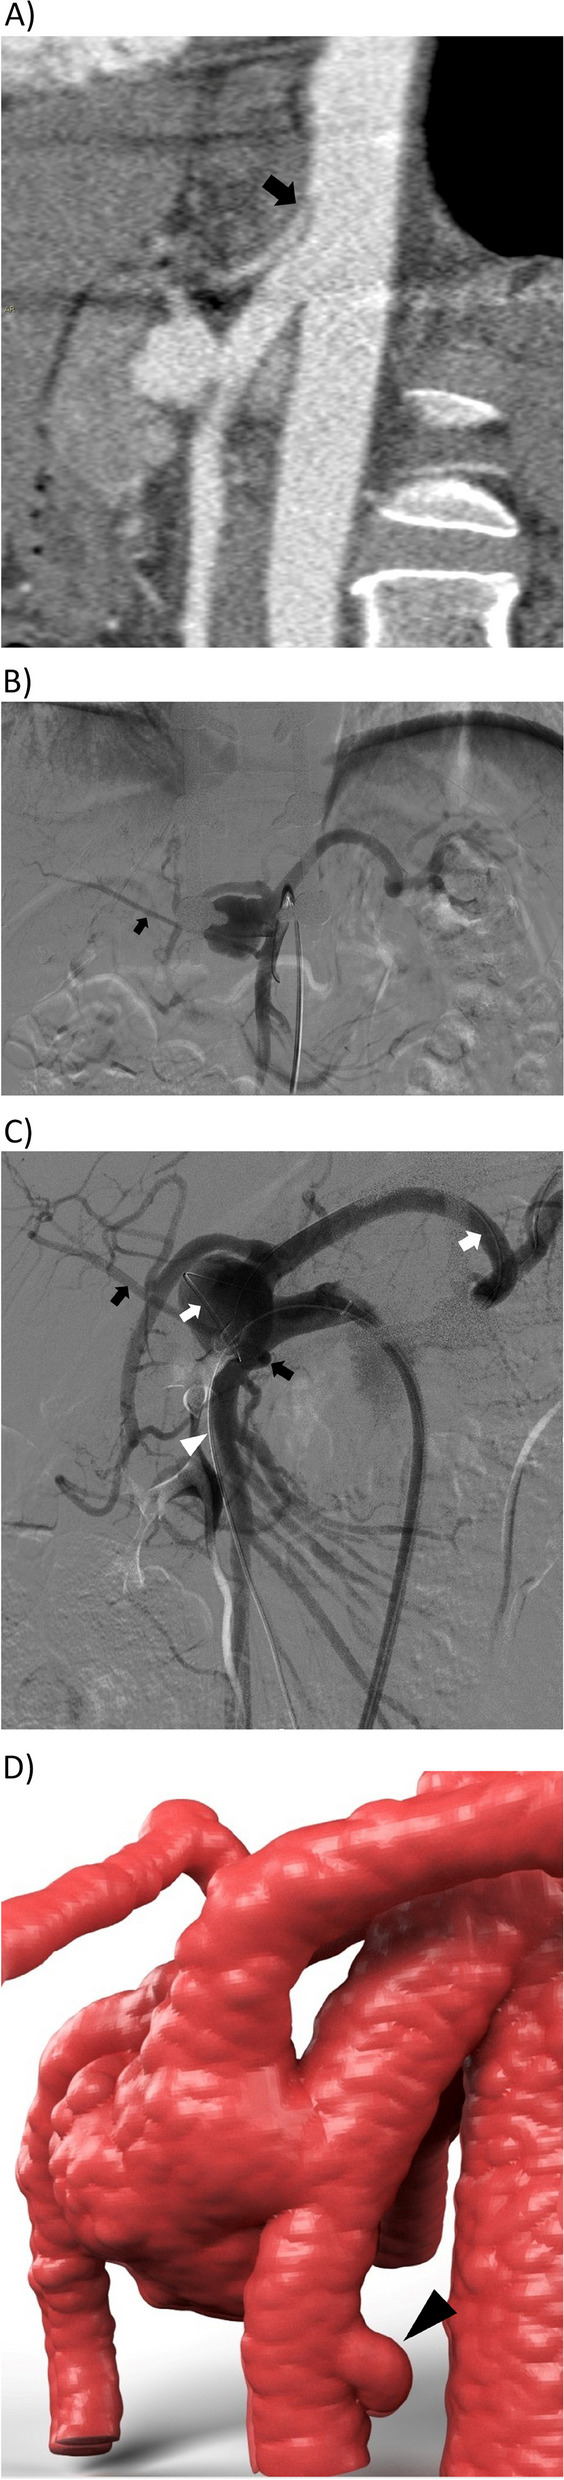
Preoperative imaging of the arch of Bühler (AOB) aneurysm. A Contrast sagittal CT-scan showing a 2.8 cm saccular aneurysm (arrowhead) without mural thrombus originating from SMA. Substantial pre-occlusive stenosis of celiac trunk (arrow) by LAM. B Pre stenting selective angiography of SMA, in AP projection (accessory right hepatic artery (black arrow)), and oblique projection C allows insight into this anatomy variant. A 0.035 “Amplatz guidewire as safety wire is stabilized in SMA (white arrowhead) while the working 0.014”micro-guidewire end in splenic artery (white arrow) after looping inside the aneurysm. A small accessory right hepatic artery (black arrow) is also present. D Its origin (arrowhead) on VR3D reconstruction was thereafter covered by Viabahn without consequences
